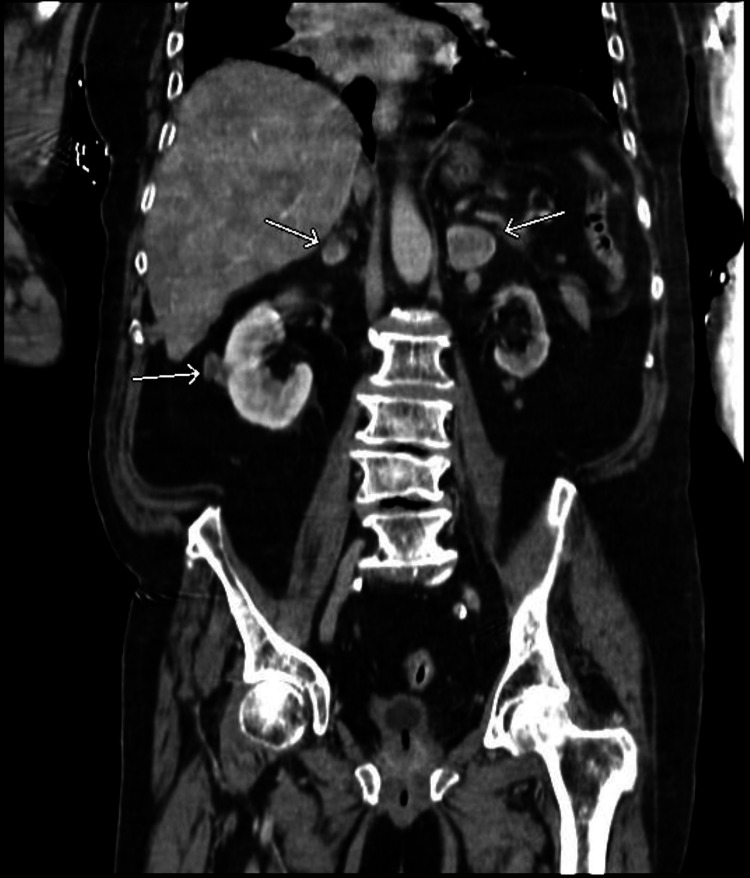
CT of the abdomen (coronal view) showing adrenal nodules and kidney nodular densities
